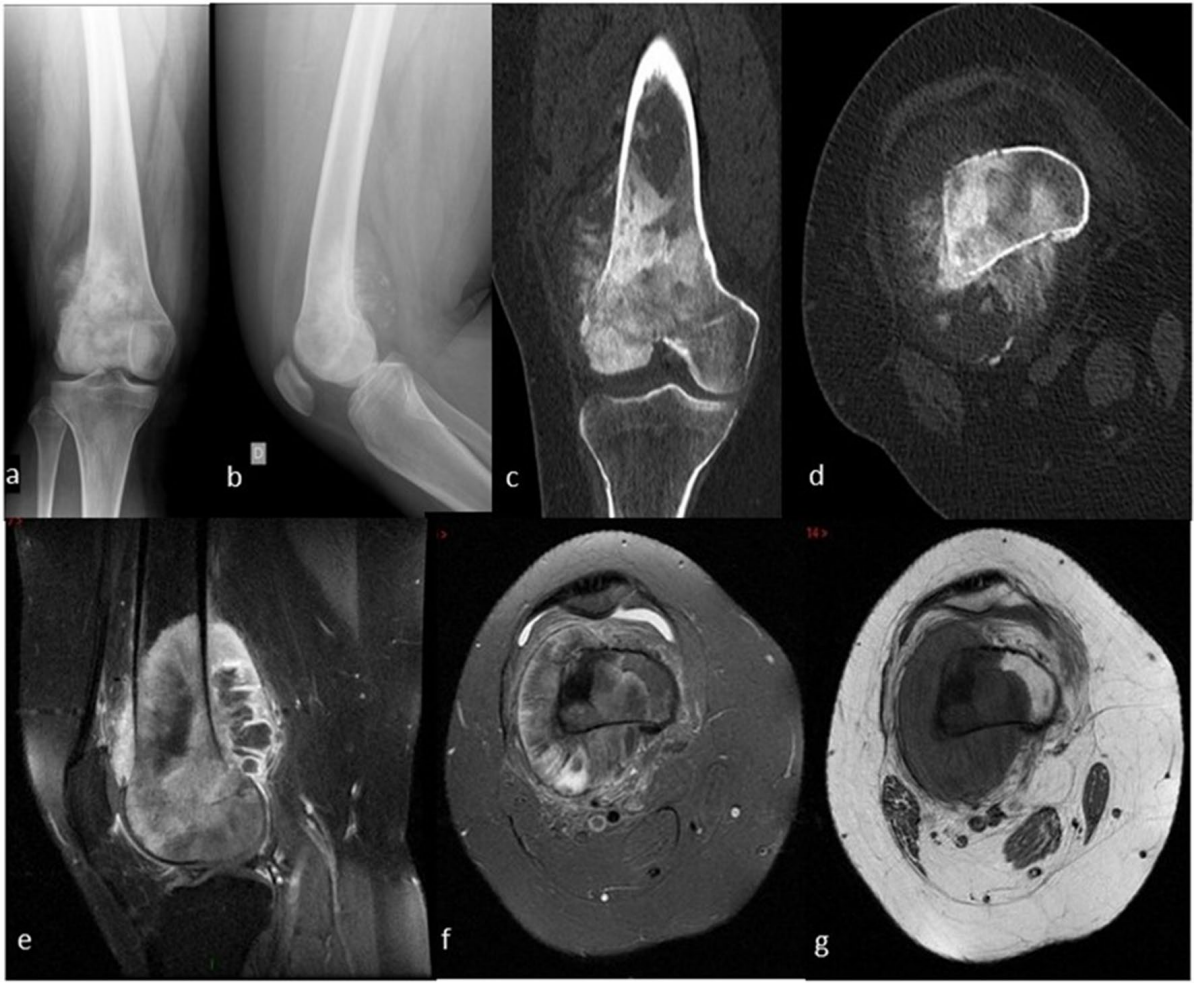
Case of osteosarcoma. A 25 year-old female, no smoker and no drinker, suffered of increasing daily inflammatory right knee pain in the last 6 months. Swelling was noticed few days ago. A tender swelling over the external part of the knee was palpable. Knee mobility was normal. (1a/b) Anteroposterior and lateral radiographs of the knee. (1c/d) Coronal and axial views on computed tomography. (1e/g) T1 sagittal view, T2 fat suppressed axial and T1 sag fat suppressed axial views on magnetic resonance imaging.
